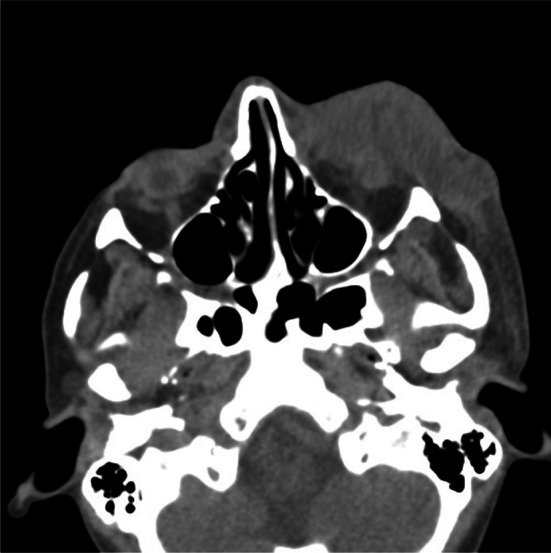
The computed tomography image shows phlegmon of the left cheek and orbital
